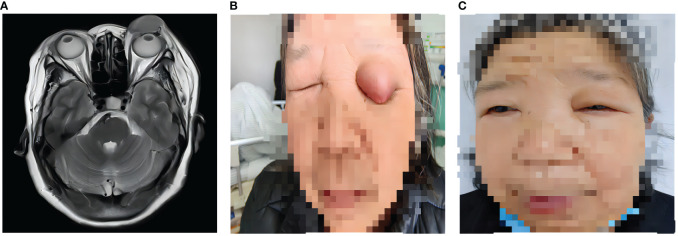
The change of ccRCC metastasis on the left eyelid. (A) MRI image of the mass on the left eyelid. (B) A painful mass on the left eyelid. (C) The mass was disappeared after 1 months of sunitinib treatment.
